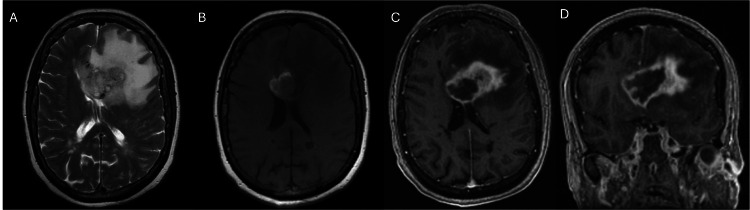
Butterfly-wing lesion on MRI. Axial T2-weighted MRI shows marked perilesional edema collapsing the frontal horns of the lateral ventricles (A). Axial T1-weighted MRI (B) reveals a small hyperintensity around the lesion, which, after contrast administration (C), avidly enhances circumferentially with a non-enhancing center. The coronal gadolinium-enhanced T1-weighted MRI (D) demonstrates the lesion with a necrotic center and peripheral contrast enhancement, crossing both sides of the midline.
